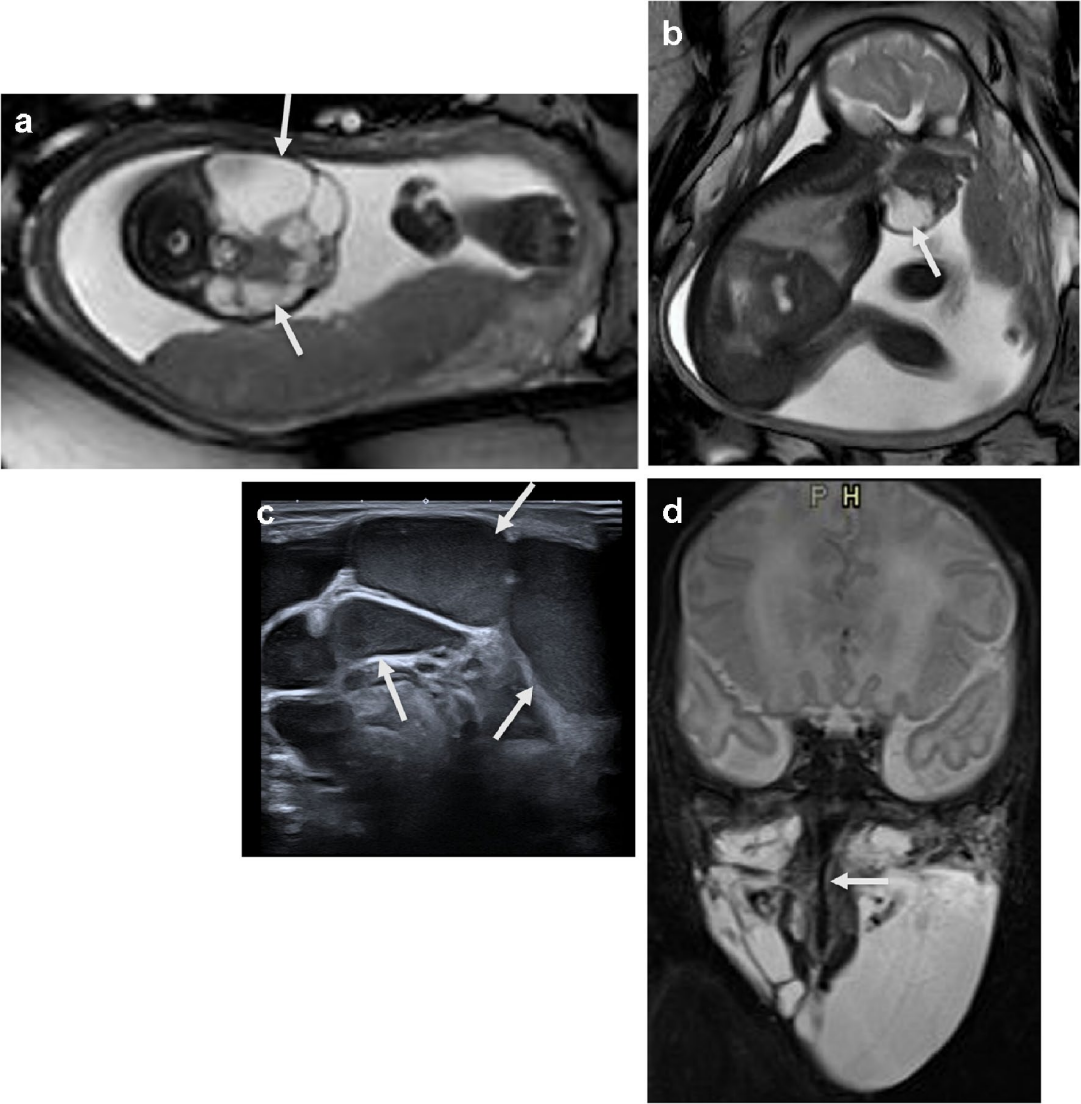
A female fetus of 31 weeks gestational age with a lymphatic malformation adjacent to the pharynx and trachea that required perinatal intervention to secure the airway. a, b Axial (a) and sagittal (b) T2-weighted magnetic resonance images demonstrate the macrocystic lymphatic malformation (*arrows*) in the submandibular region extending to the pharynx and trachea, without compression of these structures. A vaginal birth was anticipated and indeed the neonate presented no signs of airway obstruction after birth. c Ultrasound of the lymphatic malformation in the oblique plane, performed directly after term birth, demonstrates the dense fluid in several cysts, which could be hemorrhagic (*arrows*). d Postnatal coronal magnetic resonance image at day 2 shows slight compression of the trachea (*arrow*) caused by the surrounding lymphatic cysts
